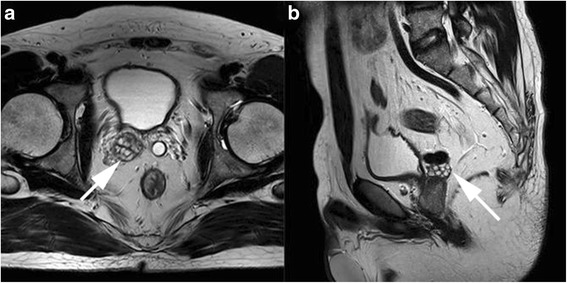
Pelvic magnetic resonance imaging (MRI) revealed left seminal vesicle aplasia combined with right ejaculatory duct cysts. a horizontal section. b sagittal section. The arrows in a and b are both pointing to the right ejaculatory duct cysts
